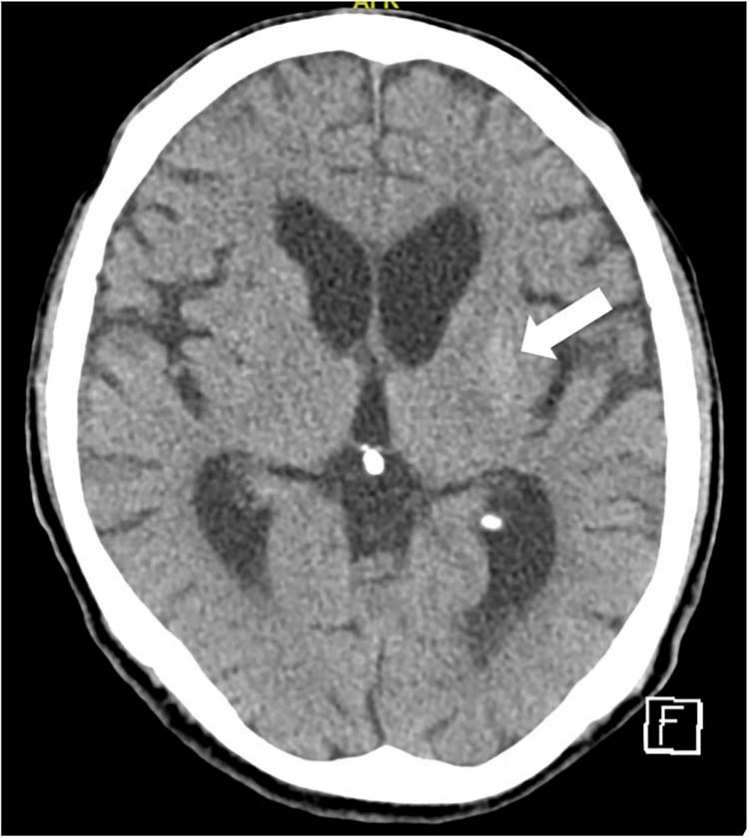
Brain CT scan showed increased density in the left basal ganglia (arrow) during patient’s second admission.
